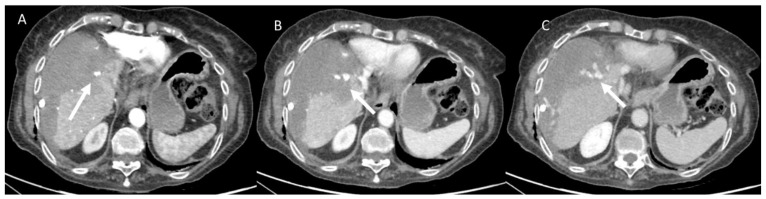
Woman 67 years at 1-day follow-up after radio frequency ablation of liver metastases. CT assessment (A) arterial phase; (B) portal phase and (C) late phase: active bleeding is present (arrow).
